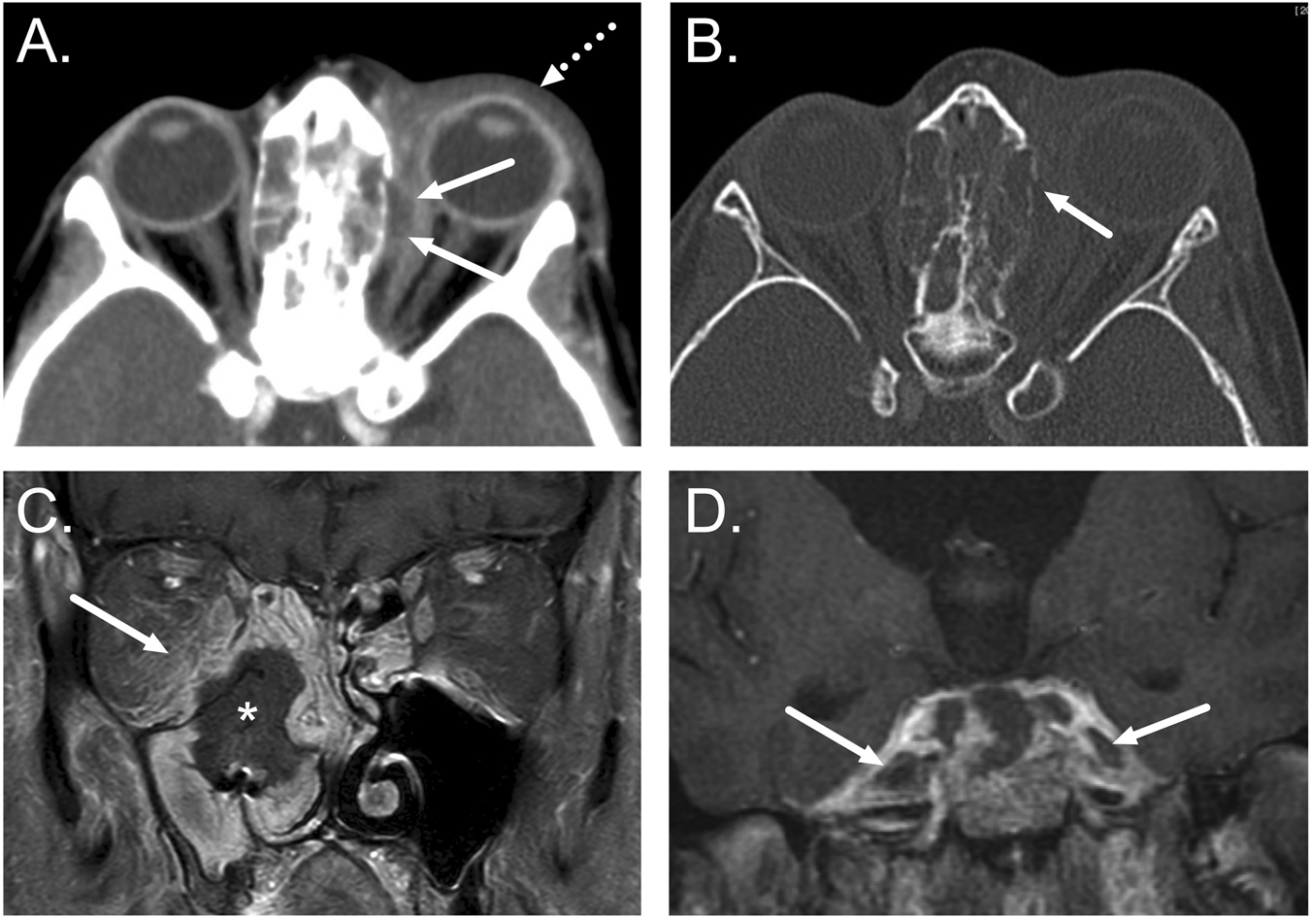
A, B Complications of acute sinusitis. Axial CT images show a subperiosteal orbital abscess secondary to ethmoiditis (arrows in A), with bony dehiscence in lamina papyracea (B). Note the preseptal swelling (dotted arrow in A). C, D Aspergillus-related AIFS in an immunocompromised patient. C Coronal post-contrast T1-weighted image demonstrates a large non-enhancing area in the right nasal cavity, maxillary sinus, and orbit (“black turbinate sign”, asterisk), along with extensive infective changes in the orbit (arrow). D Coronal post-contrast T1-weighted image from another patient shows bilateral cavernous sinus thrombosis as filling defects (arrows)
